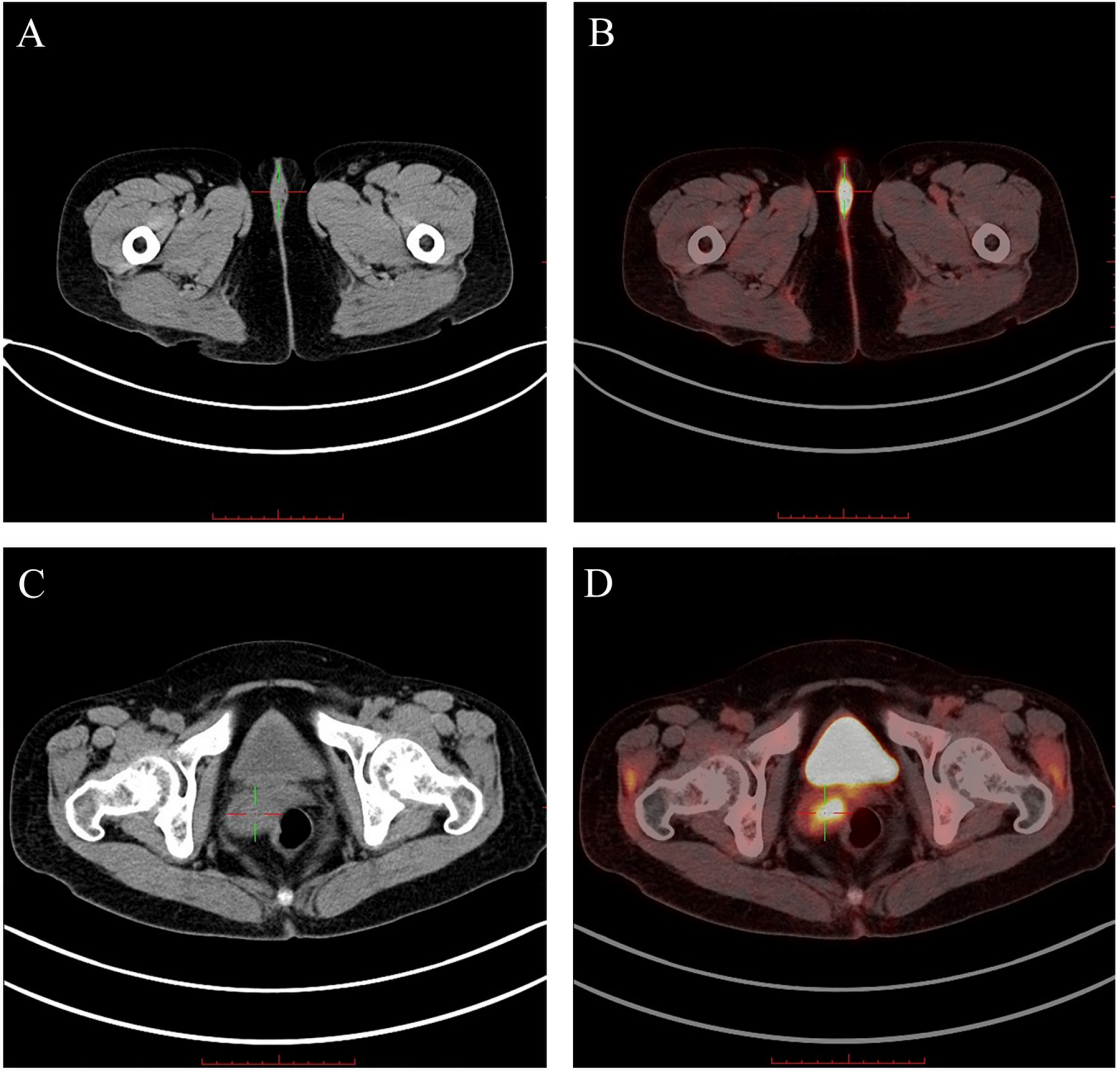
The PET-CT scans obtained from case 1 ("+" presents the vulvar/cervical lesion): (A) The CT showed a low-density nodular shadow in the vulva. (B) The PET-CT showed significantly increased 18F-FDG metabolism in the vulvar lesion. (C) The CT showed an uneven density mass shadow in the cervix. (D) The PET-CT showed significantly increased 18F-FDG metabolism in the cervical lesion.18F-FDG, 18F-fluorodeoxyglucose.
